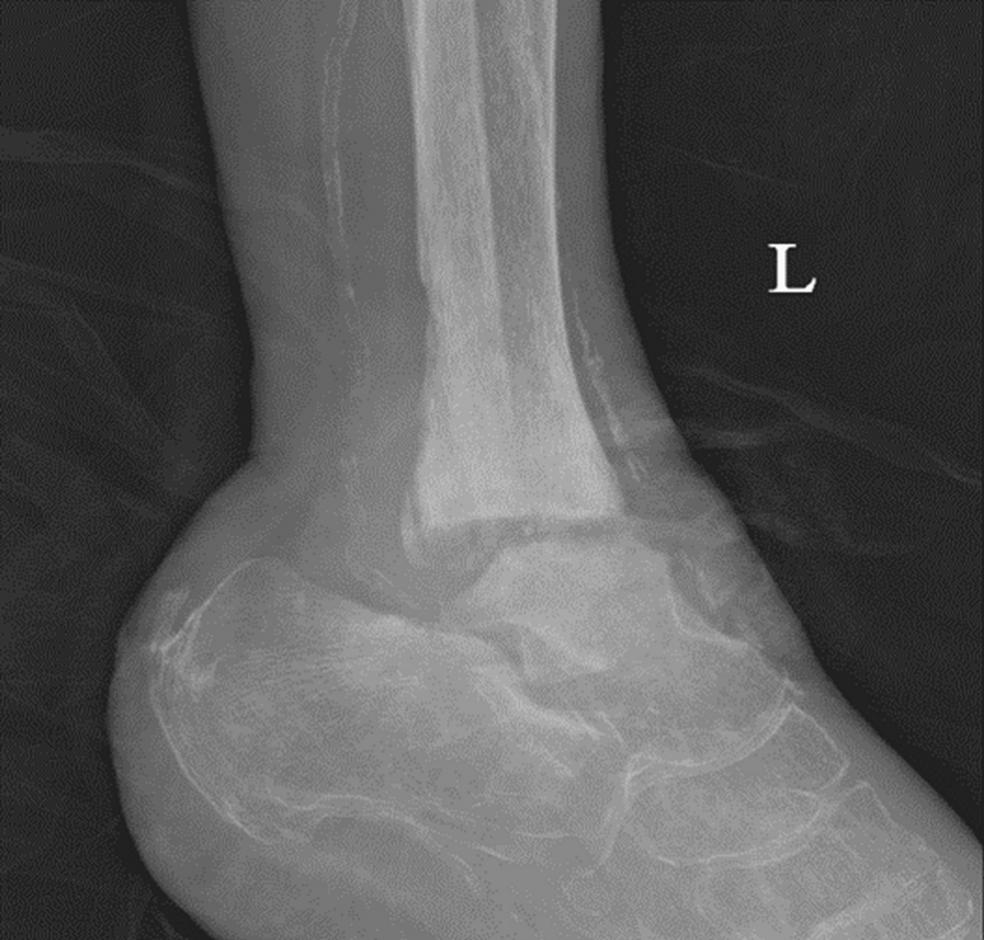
Lateral X-ray of the left ankle/foot
